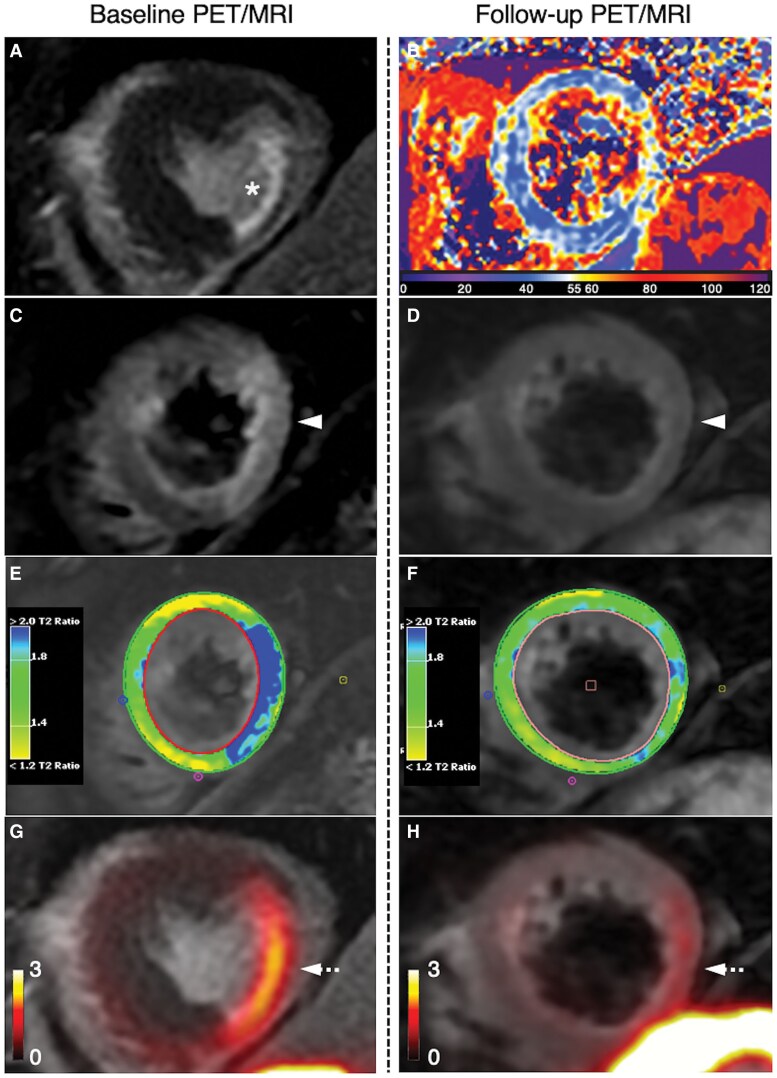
Tracking myocardial inflammation using ^68^Ga-DOTATATE PET/MRI at baseline vs. 3-month follow-up. Baseline PET/MRI (left): *A*) LGE-MRI showing subendocardial MI (white asterisk) in the mid inferolateral segment, with corresponding *C*) high signal (white arrowhead) on T2-weighted imaging, and *G*) increased 68Ga-DOTATATE uptake (white dashed arrow). *E*) Colour map shows myocardium-to-muscle T2 ratio. Follow-up PET/MRI (right): *B*) T2-mapping; *D*) resolution of oedema on T2-weighted imaging; *F*) myocardium-to-muscle T2 ratio; *H*) residual 68Ga-DOTATATE uptake indicating ongoing macrophage activity (white dashed arrow). Reproduced from Ćorović *et al.*, open access under Creative Commons CC-BY 4.0.^49^
